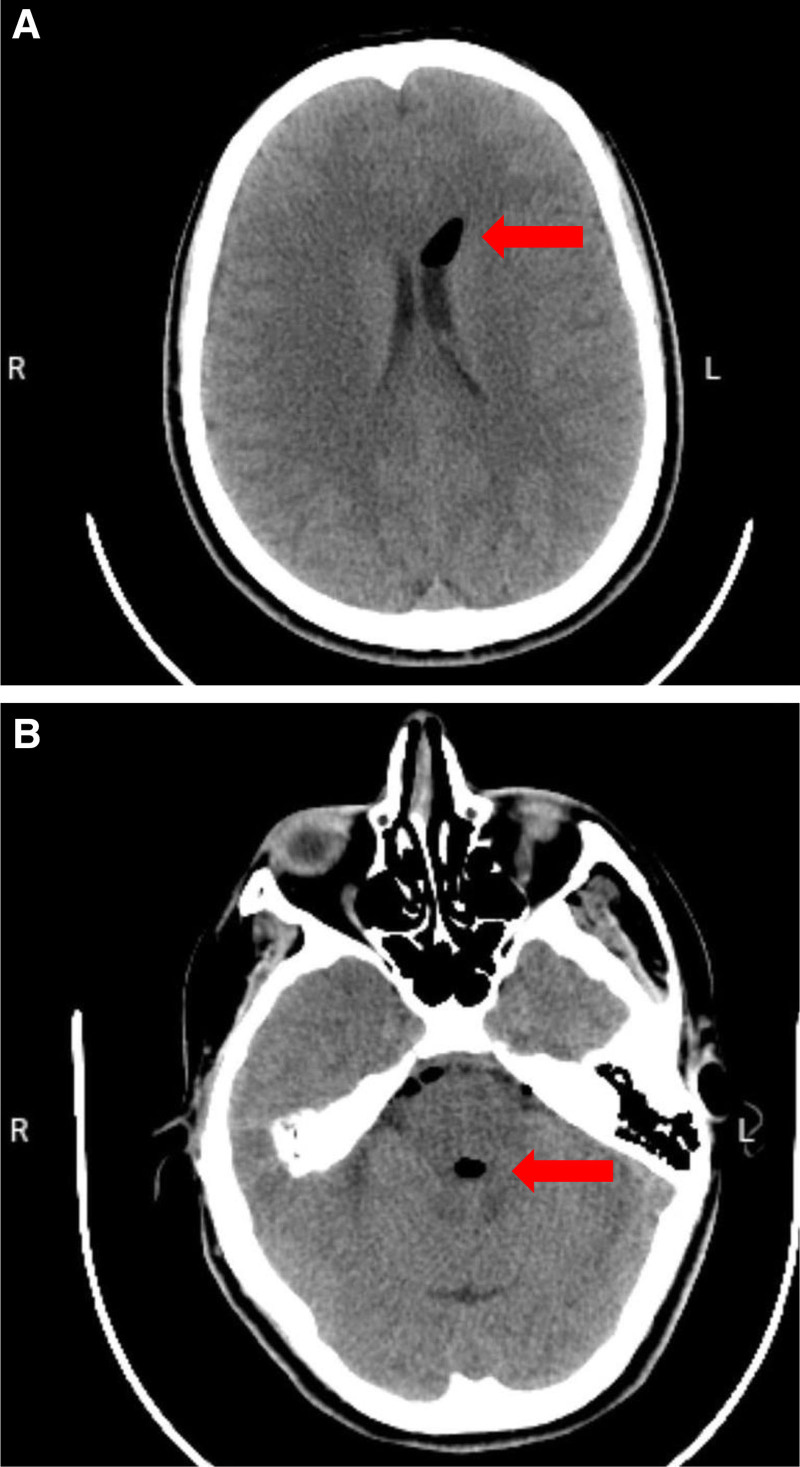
(A) Axial non-contrast CT scan of the brain showing a well-defined, crescent-shaped hypodense area (red arrow) consistent with intracranial air within the frontal horn of the left lateral ventricle, indicative of pneumocephalus. (B) Initial axial non-contrast CT scan of the brain showing pneumocephalus with air collection in the prepontine cistern (red arrow), consistent with peripontine pneumocephalus. CT = computed tomography.
